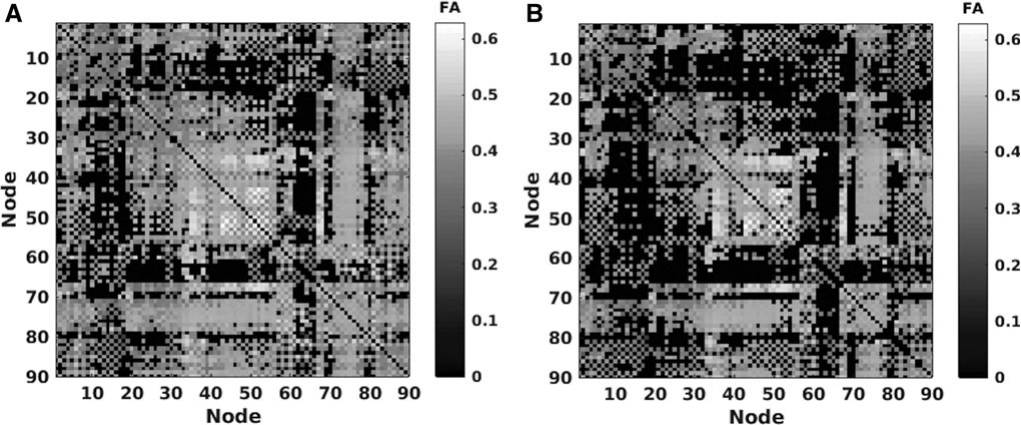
An example of the conservative threshold added to all dMRI connectivity matrices. (A) FA connectivity matrix for one participant before thresholding. (B) After a conservative threshold of five streamlines was applied to FA for the same participant. dMRI, diffusion-weighted magnetic resonance imaging; FA, fractional anisotropy.
